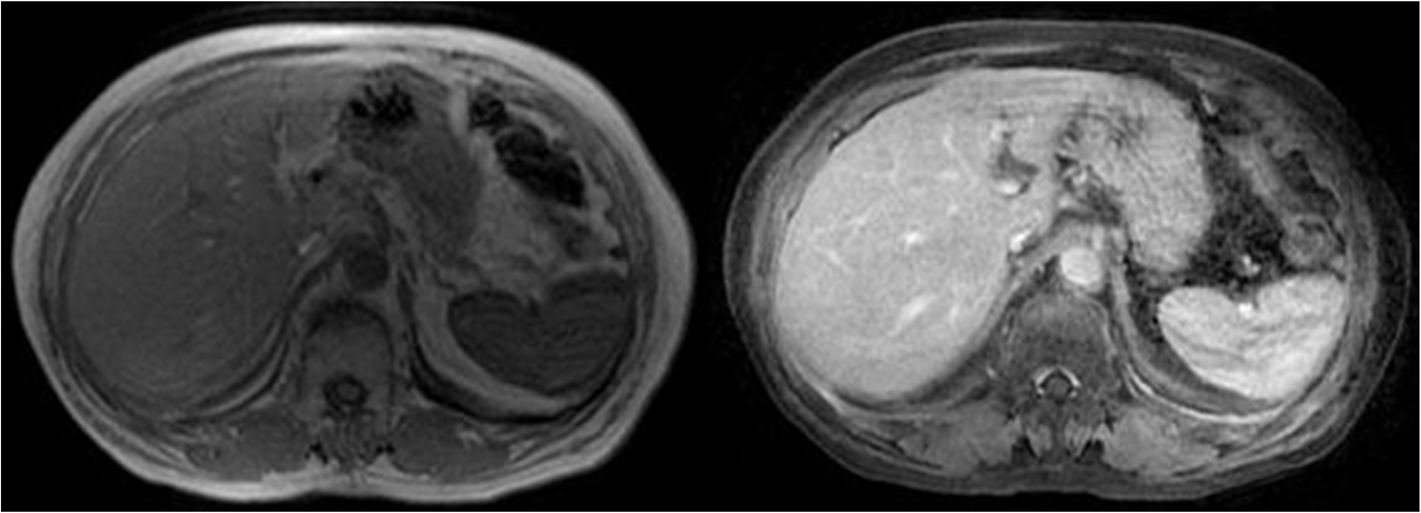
The MRI performed at 2 months showed the disappearance of the lesions
